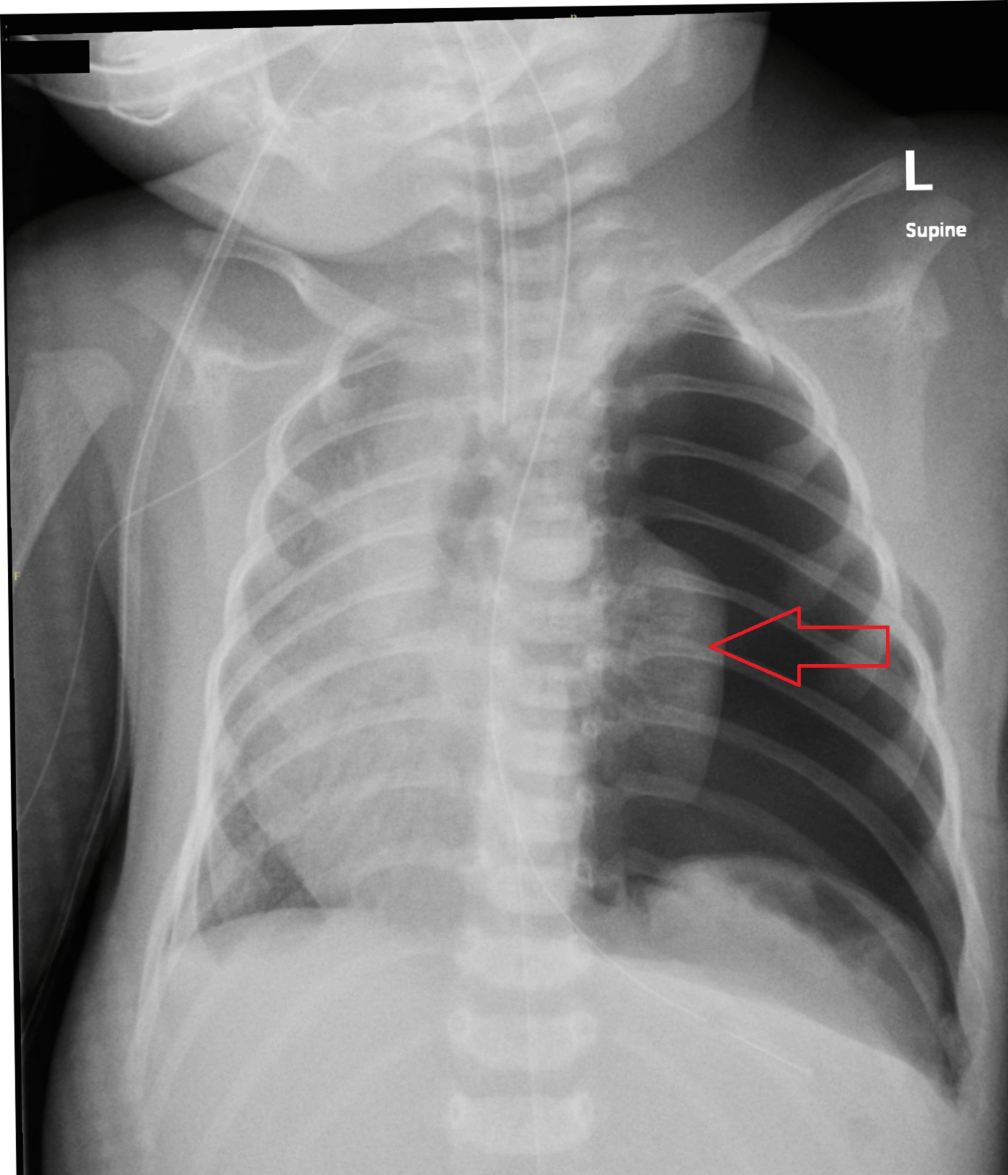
Chest X-ray performed after thoracoscopic repair of a left congenital diaphragmatic hernia revealed hypoplastic left lung (arrow).
